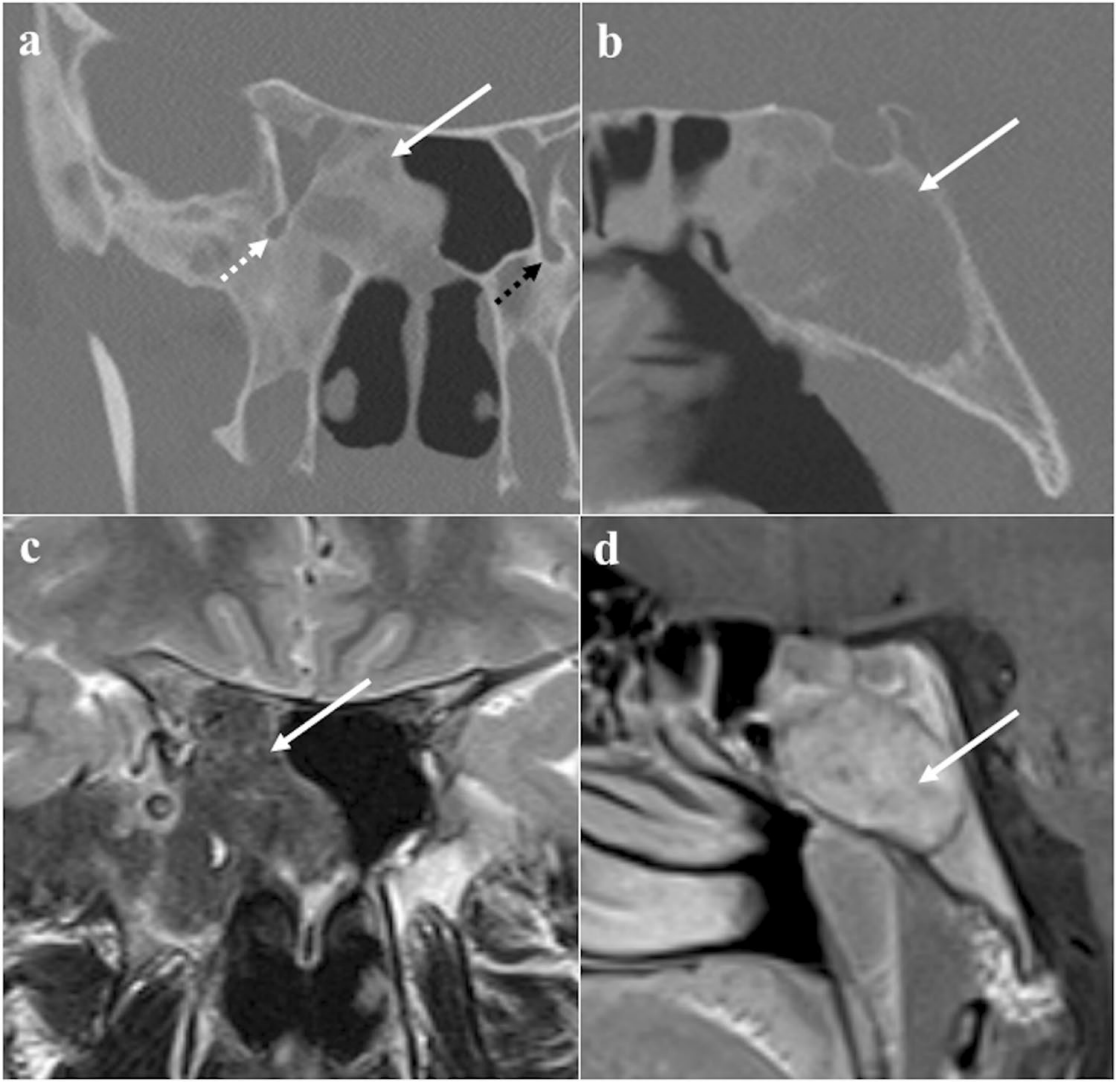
Fibrous dysplasia in a 20-year-old male patient with right atypical trigeminal neuralgia. Coronal CT image (a) reveals an expansile lesion in the middle cranial fossa extending into the right sphenoid sinus, pterygoid plates, sphenoid wings, and parietal bone with a “ground glass” appearance representing fibrous tissue (white arrow). Notice the narrowing of the right foramen rotundum (white dotted arrow) compared to the contralateral (black dotted arrow). Sagittal CT image (b) shows expansion of the clivus (arrow). At MRI, the lesion shows low SI on coronal T1W image (c, arrow) and highly inhomogeneous enhancement on sagittal T1W CE image (d, arrow)
